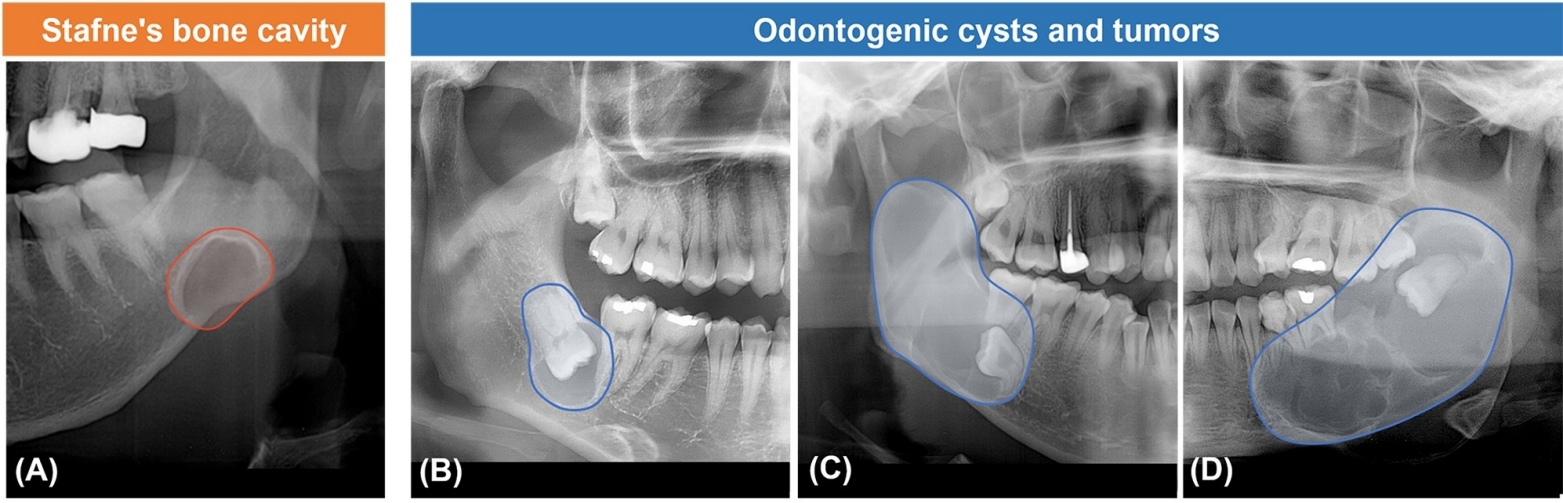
Cropped panoramic radiography of the individual lesion. (A) Stafne’s bone cavity, (B) Dentigerous cyst, (C) Odontogenic keratocyst, (D) Ameloblastoma.
